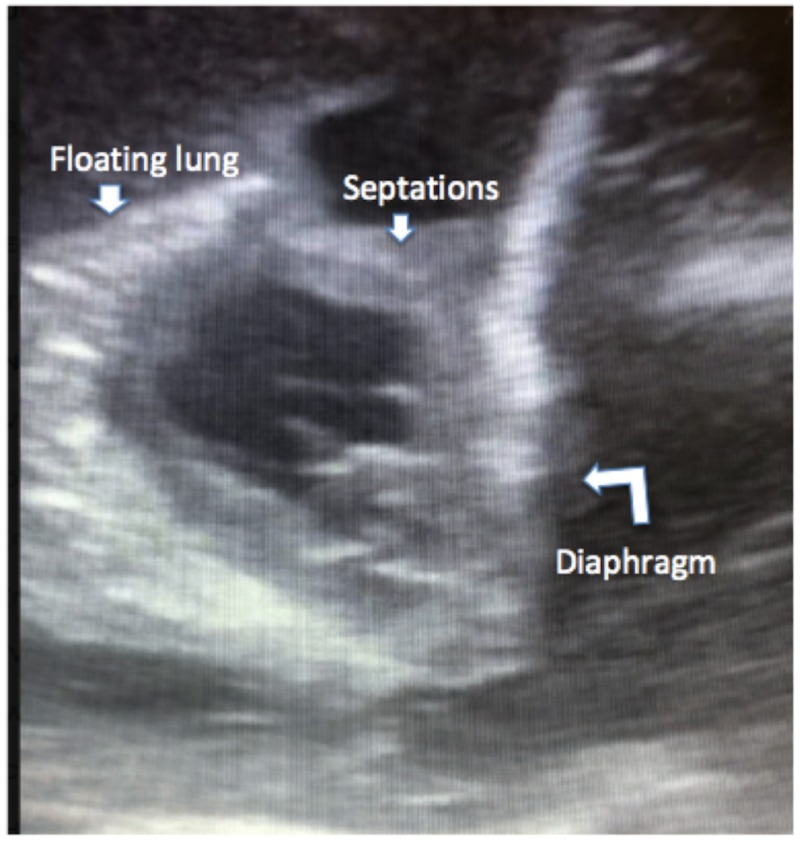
Ultrasound of the right lung Bedside ultrasound of the right lung showing complex fluid collection with multiple septations and lung ‘floating’ in pleural fluid
